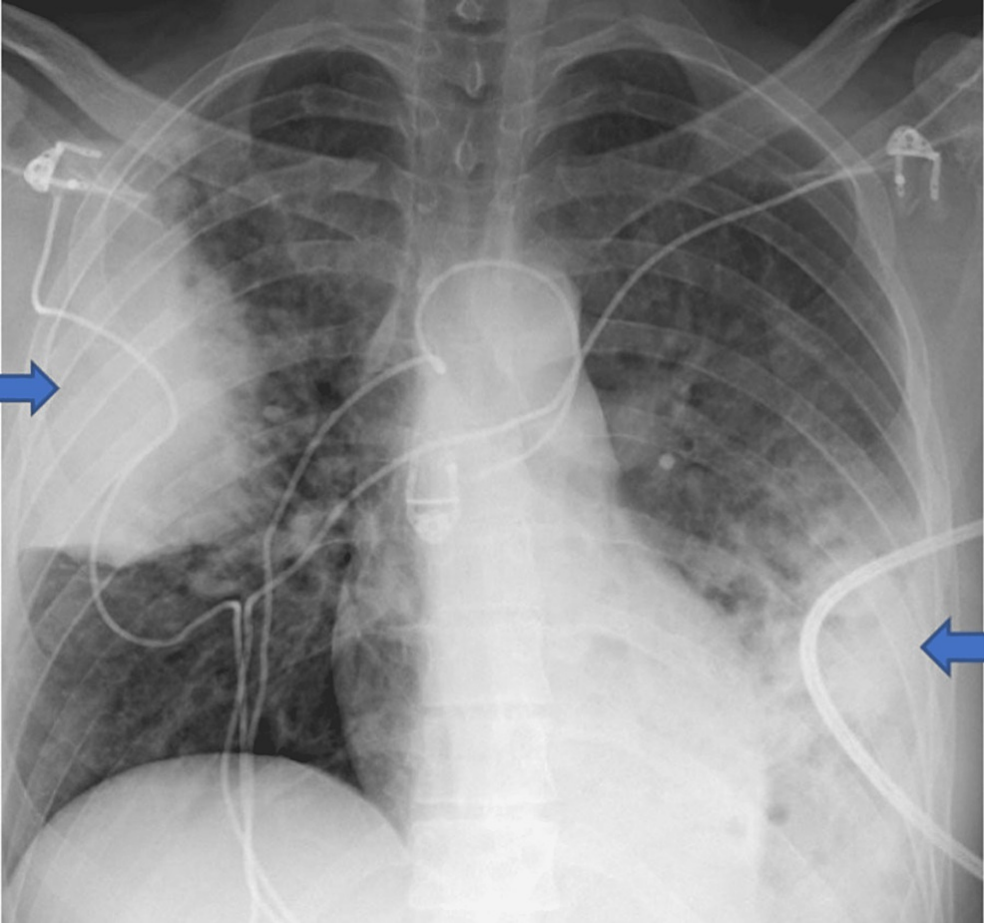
Chest X-ray showing consolidation Blue arrows show right and left lung consolidation.
